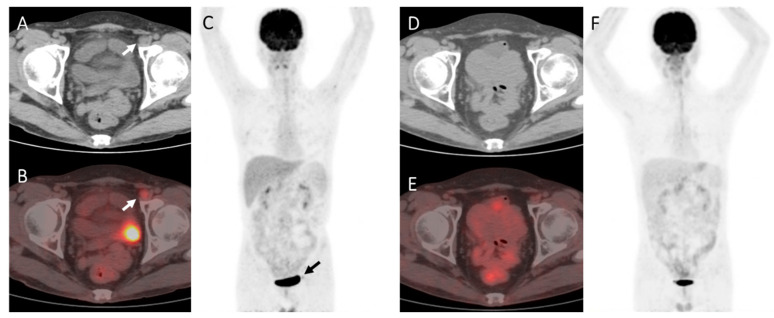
A case of B-Lymphoblastic lymphoma of the pancreas and bones in a 43-year-old male. The patient was treated with chemotherapy and achieved complete remission. The patient then received allogeneic SCT and underwent ^18^F-FDG PET-CT 1.5 months after transplantation for assessment. (A–C): PET showed an FDG-avid pelvic lymph node (arrow), about 1.3 * 1.1 cm^2^ in size, SUVmax3.5 (DS = 4). (D–F): A follow-up PET imaging was carried out 3 months later with no treatment, which showed the FDG-avid foci disappeared. Therefore the post-SCT PET at 1.5 months was determined as a false positive result.
